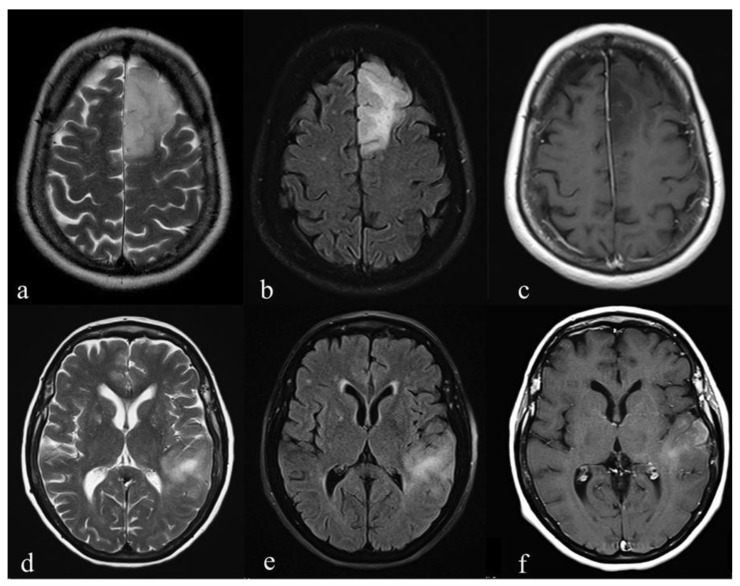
Pre-surgical conventional MR images of two patients with Grade III astrocytoma: (a–c) T2-weighted, fluid-attenuated inversion recovery images (FLAIR) and T1-weighted post-gadolinium images of an IDH-mutant (Mut) tumor show a lesion on the left frontal lobe. The borders are well-defined on T2-weighted (a) and FLAIR (b) images. No areas of T1-shortening are evident after IV contrast medium administration; (d–f) T2-weighted, FLAIR and T1-weighted post-gadolinium of an IDH-wild type (Wt) tumor show a hyperintense T2-weighted (d) and FLAIR (e) lesion on the left temporal lobe. Ill-defined borders (d,e) and a blurred contrast enhancement are detected (f). Scale bar: 5 cm.
